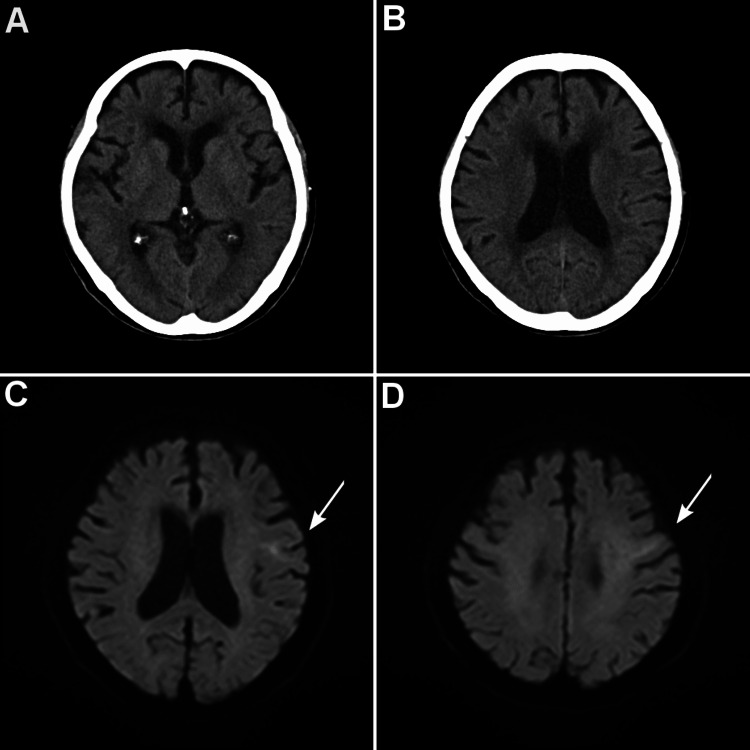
Initial Head CT and MRI just after intravenous thrombolysis, demonstrating an infarct in the left precentral gyrus. Head CT scan (A, B). There were no apparent early ischemic lesions—axial diffusion weight imaging (DWI) (C, D). The arrow indicates an infarction.
